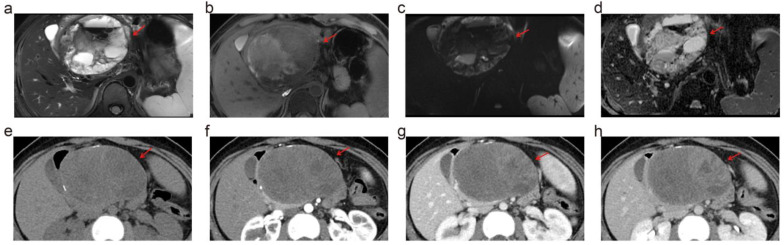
Solid pseudopapillary neoplasm in a 28-year-old woman. 5.0 T MRI ((a) for T2WI and (b) for T1WI) demonstrated the complex infrastructure of the tumor, including multiple septa and fluid–fluid levels within a portion of the lesion suggestive of intratumoral hemorrhage. The peripheral solid tumor component was hyperintense at DWI (c) and hypointense at the ADC map (d). These signs were highly suggestive of solid pseudopapillary neoplasm. On the contrary, peripheral eggshell-like calcification and a complex solid-cystic appearance with heterogeneous enhancement at multiphasic enhanced CT led to a misdiagnosis of mucinous neoplasm with malignant transformation at MDCT. (e–h) show the MDCT images in the plain scan, arterial phase, portal venous phase, and delayed phase. At pathologic analysis, this lesion was diagnosed as a solid pseudopapillary neoplasm. The red arrows refer to the lesion.
